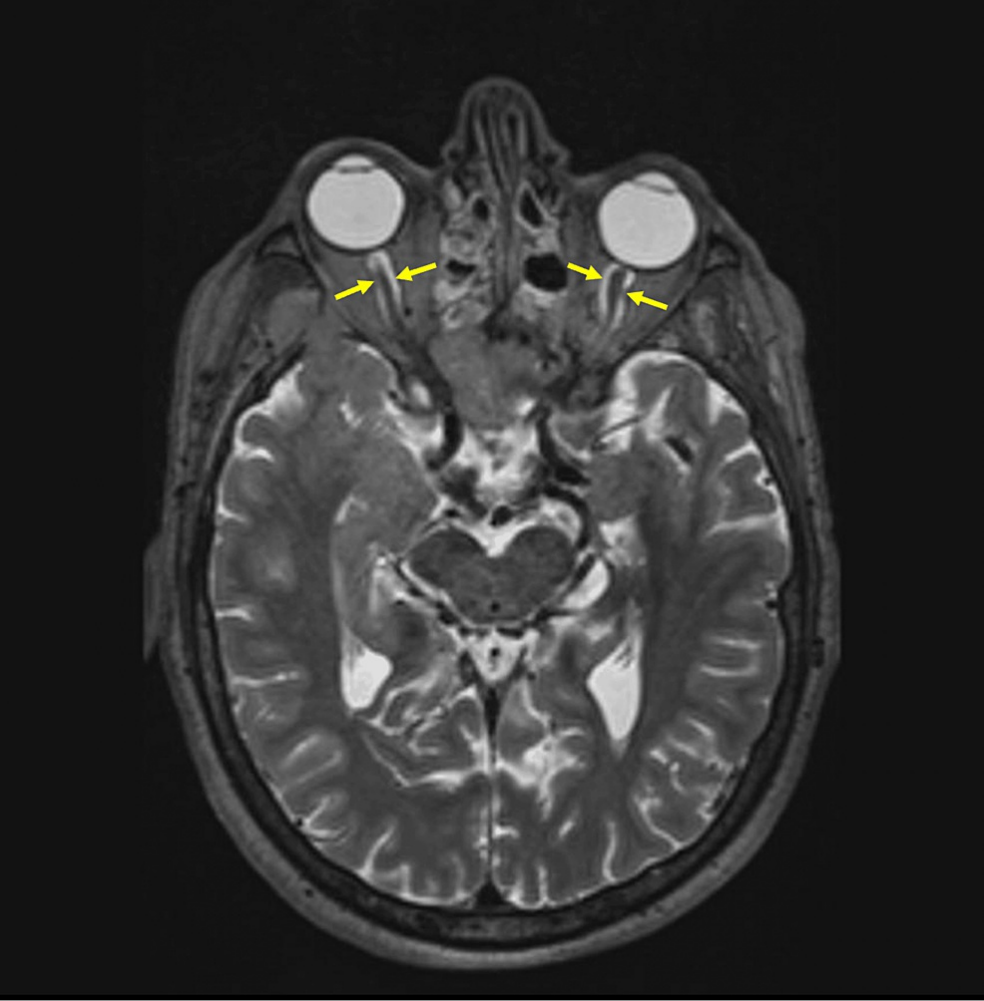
Increased fluid in the optic nerve sheath on T2-weighted magnetic resonance imaging (indicated by yellow arrows).
